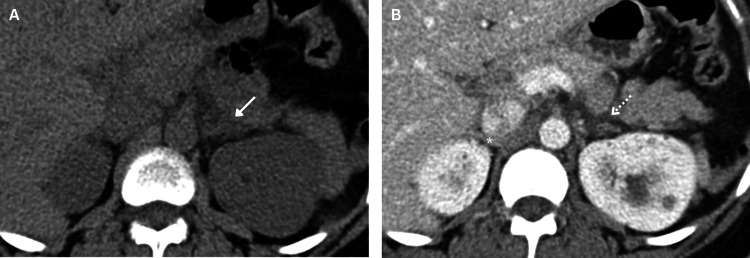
Initial CT (unenhanced and contrast-enhanced phases) demonstrating left adrenal hemorrhagic infarction. (A) Unenhanced CT shows subtle enlargement of the left adrenal gland (maximum limb thickness: 9 mm) (arrow) with increased attenuation on the left (~50 HU) compared with the right adrenal gland (maximum limb thickness: 3 mm; ~35 HU) (not shown). (B) Portal venous phase shows mild hypoenhancement of the left adrenal gland (dotted arrow) compared with the contralateral side (asterisk), with heterogeneous attenuation in the left adrenal gland (~22-110 HU), including early low-attenuation areas (~22 HU) and focal relatively hyperenhancing components (up to ~110 HU), while the contralateral adrenal demonstrates homogeneous enhancement (~100 HU).
